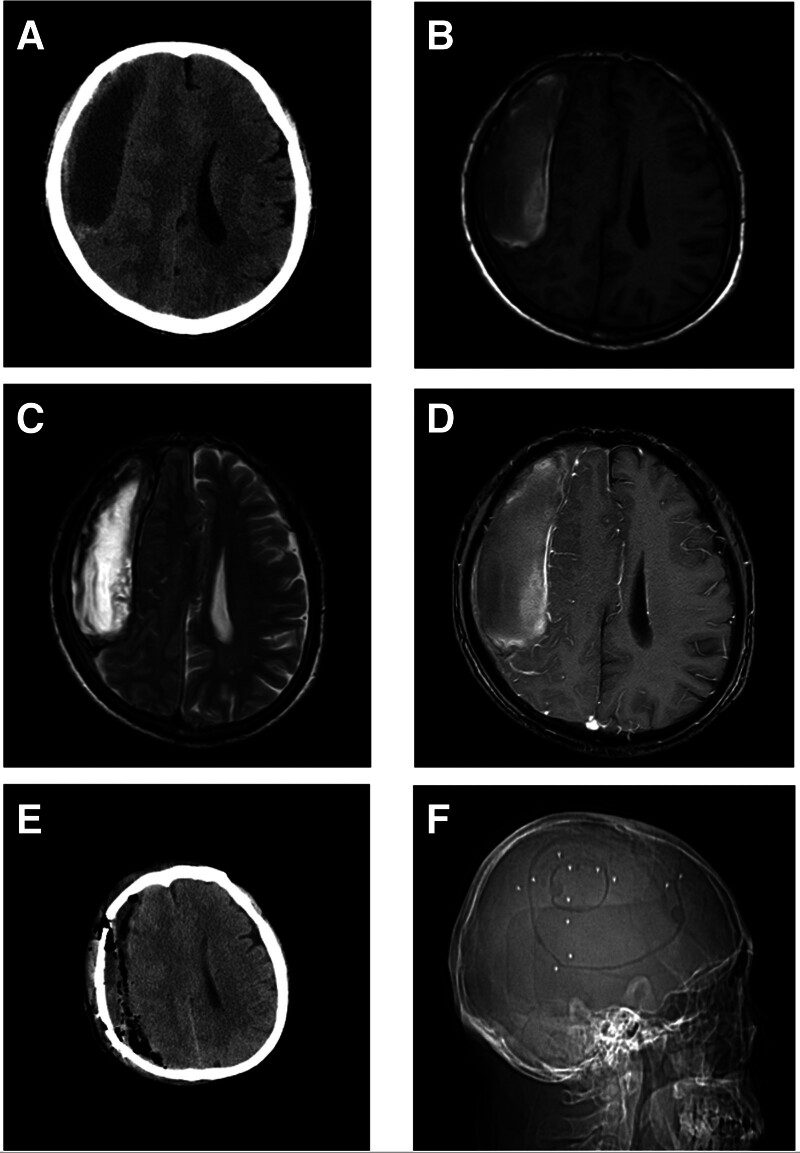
Imaging examination results of the patient. (A) The preoperative CT scan revealed a low-density subdural mass located in the right frontal, temporal, parietal, and occipital regions, causing a midline shift towards the contralateral side. (B) The preoperative T1 sequence revealed mixed signal shadows, predominantly exhibiting high signal intensity in the subdural region of the right frontal, temporal, parietal, and occipital lobes. (C) The preoperative T2 sequence revealed hypointense shadows between the lesion and the cerebral cortex, with no apparent edema signal surrounding the lesion. (D) No significant enhancement was detected within the lesion on the preoperative MR enhancement scan, with linear enhancement shadows noted along the lesion’s periphery bordering the cerebral cortex. (E) The postoperative CT scan indicated the clearance of the right subdural hematoma and a reduction in midline structural deviation. (F) The postoperative CT localization film revealed both a small bone window craniotomy shadow and a large bone flap craniotomy shadow.
